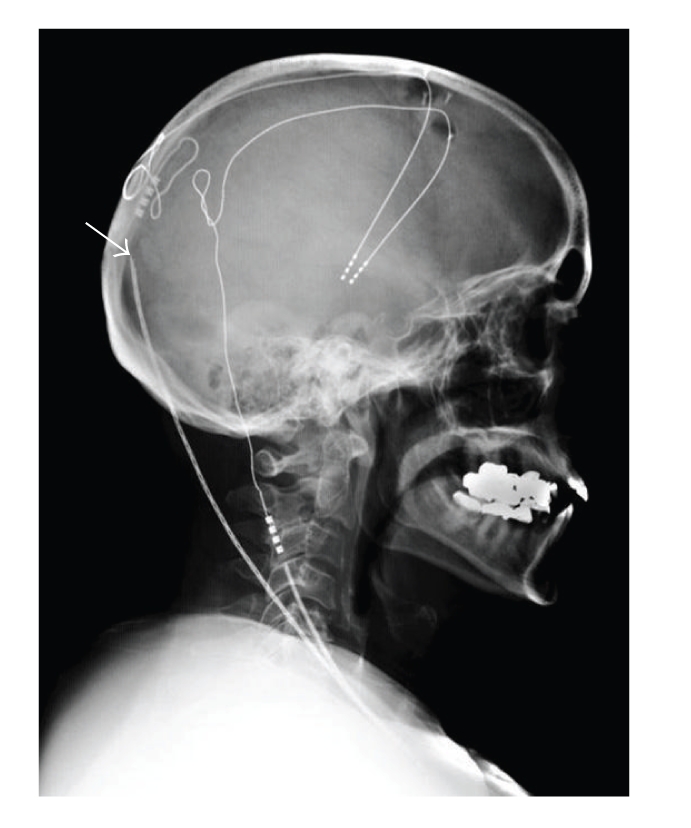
Lateral X-ray showing a new discontinuity of the right extension lead over the skull at the connection to the right DBS electrode. Note the braided appearance of the right extension lead.
